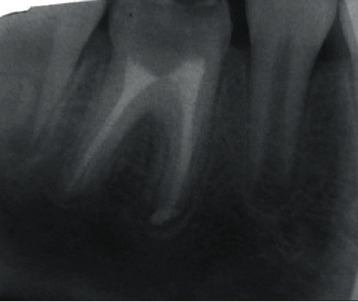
Periapical radiograph after 20 months: total regression of the apical radiolucency.
